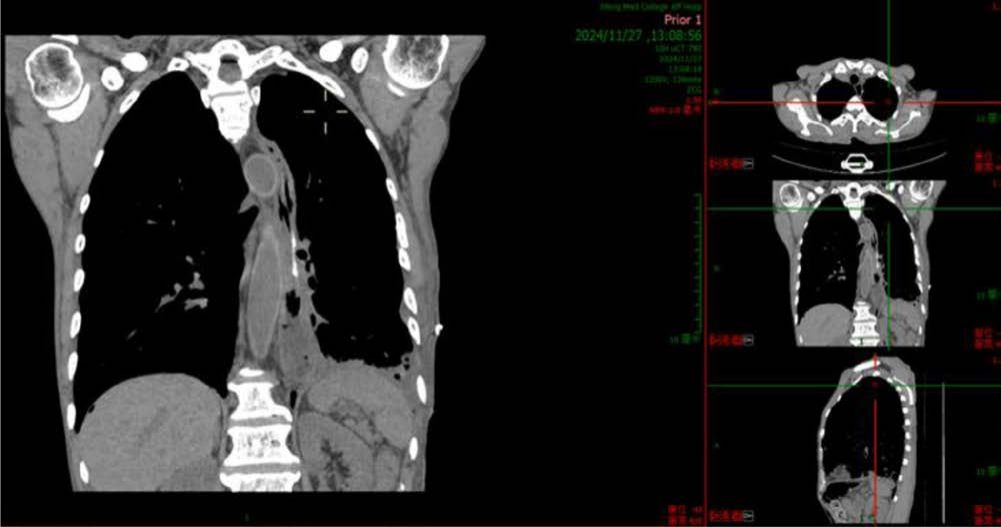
November 28, 2024, follow-up CT demonstrated postoperative changes from diaphragmatic hernia repair and partial small bowel resection, along with prior esophagectomy, with no evidence of diaphragmatic hernia recurrence. CT = computed tomography.
